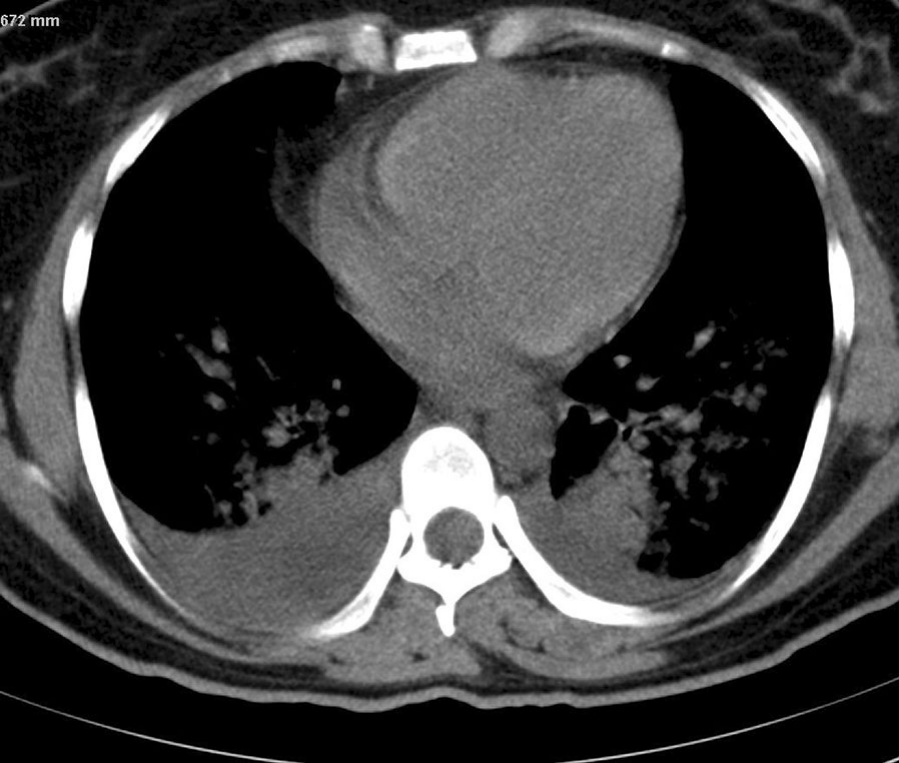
The same patient in Fig. 1 with bilateral pleural effusion is seen in CT scanning of the chest
